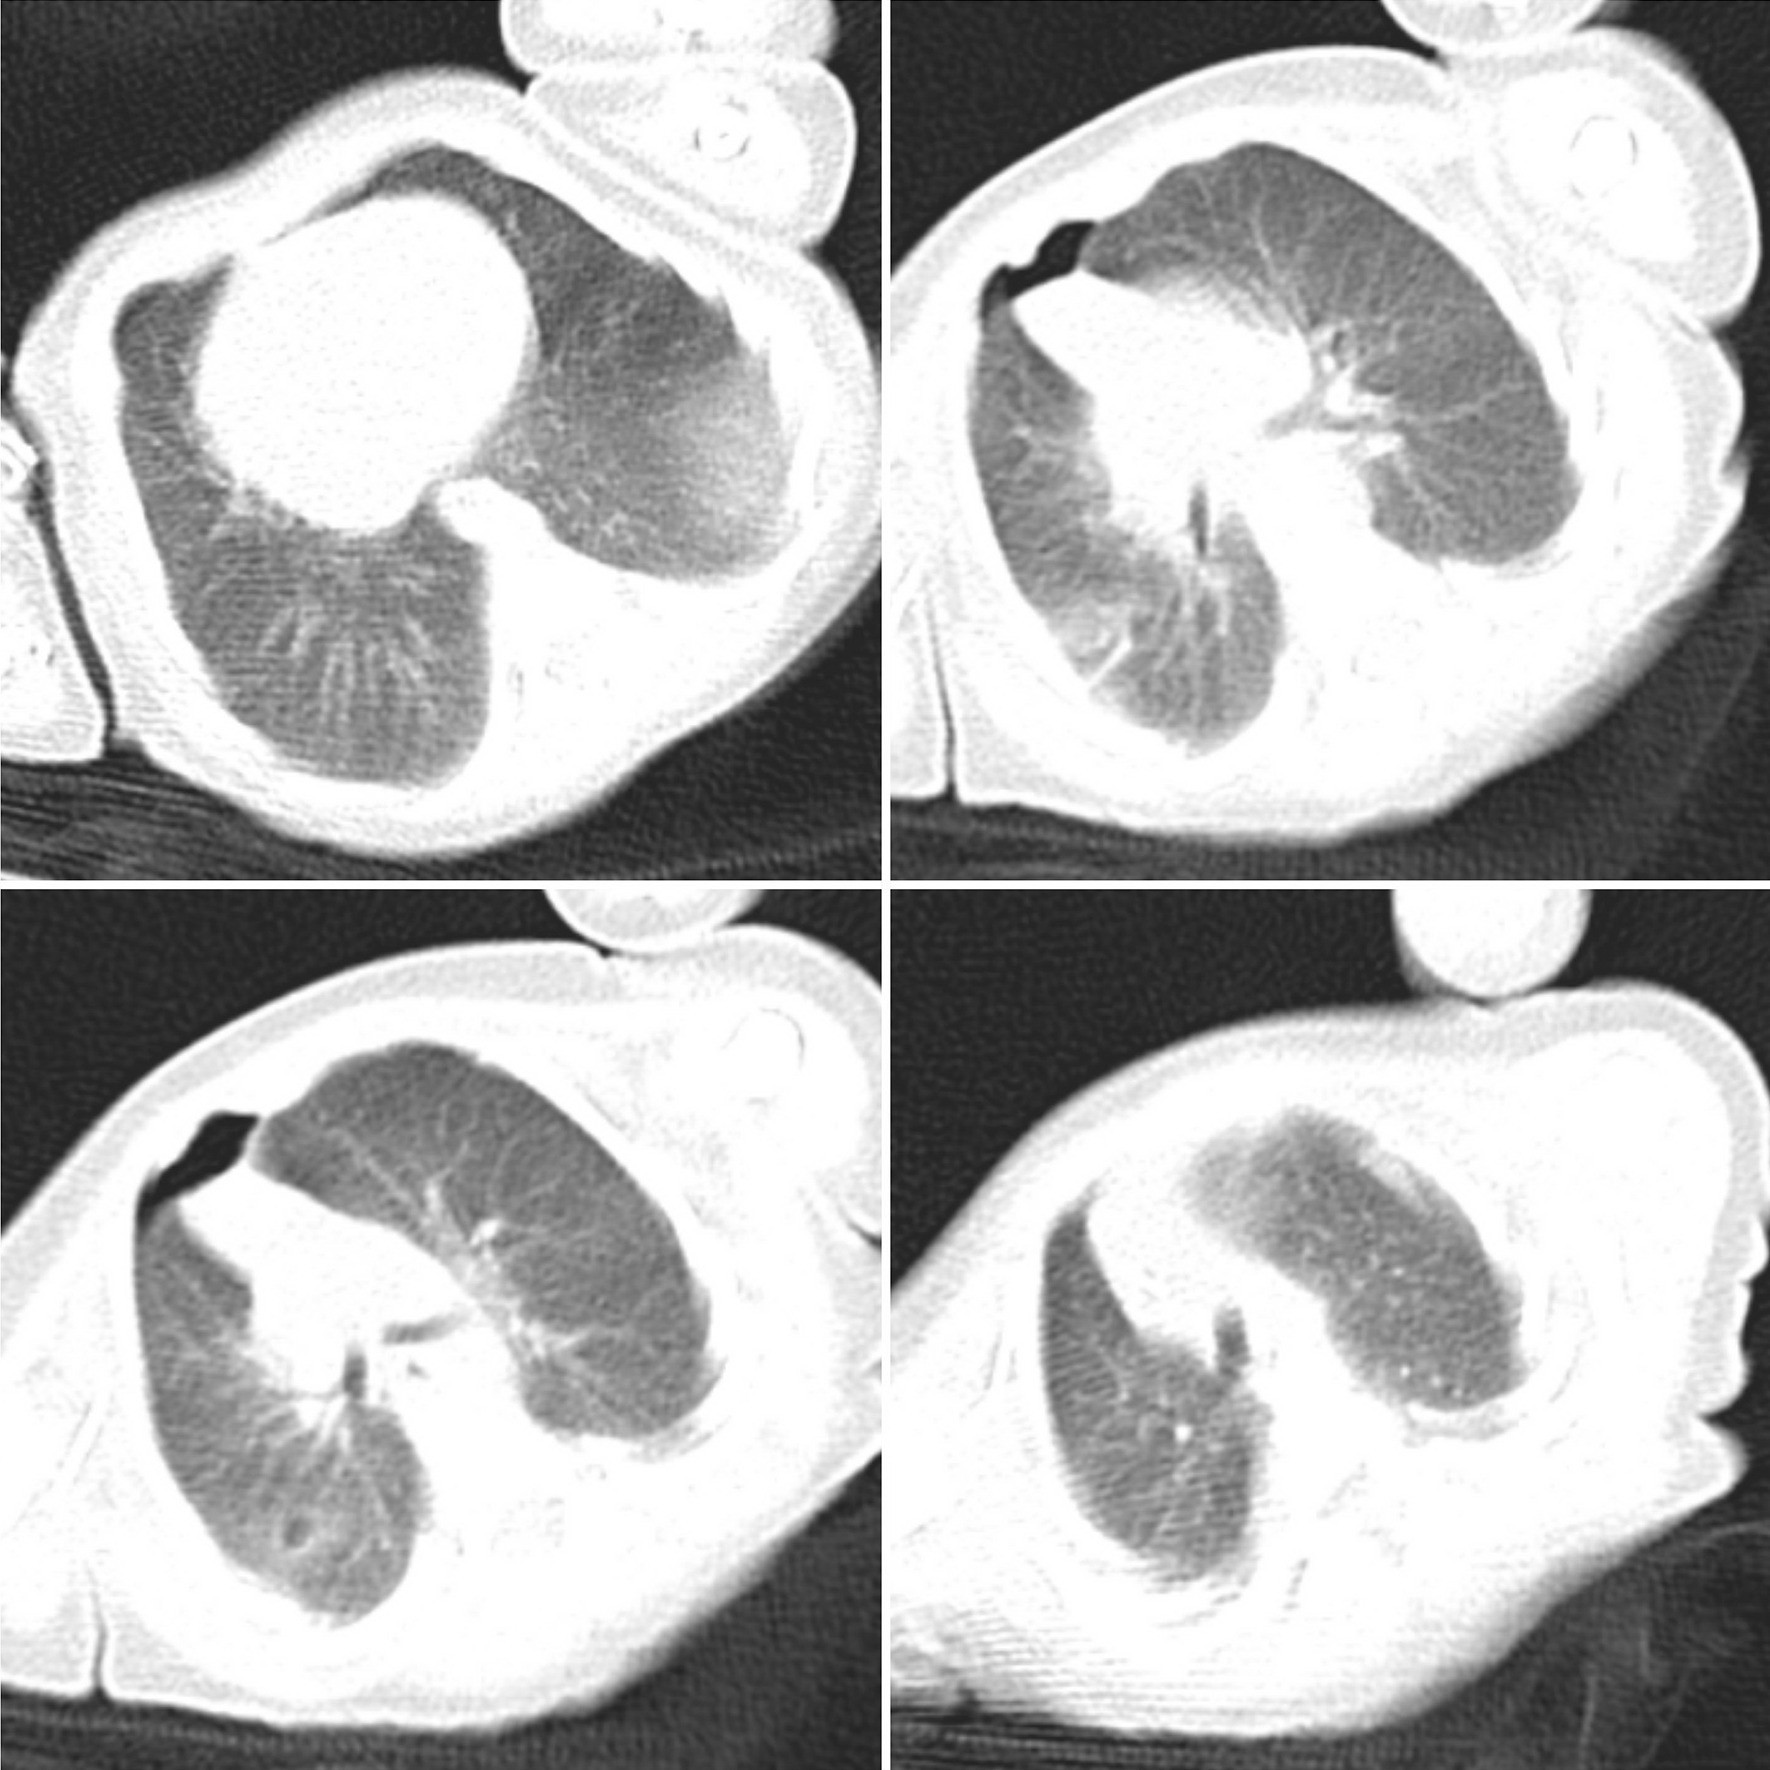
Chest computed tomography of the baby obtained on the ninth day of life showing bilateral patchy shadows
